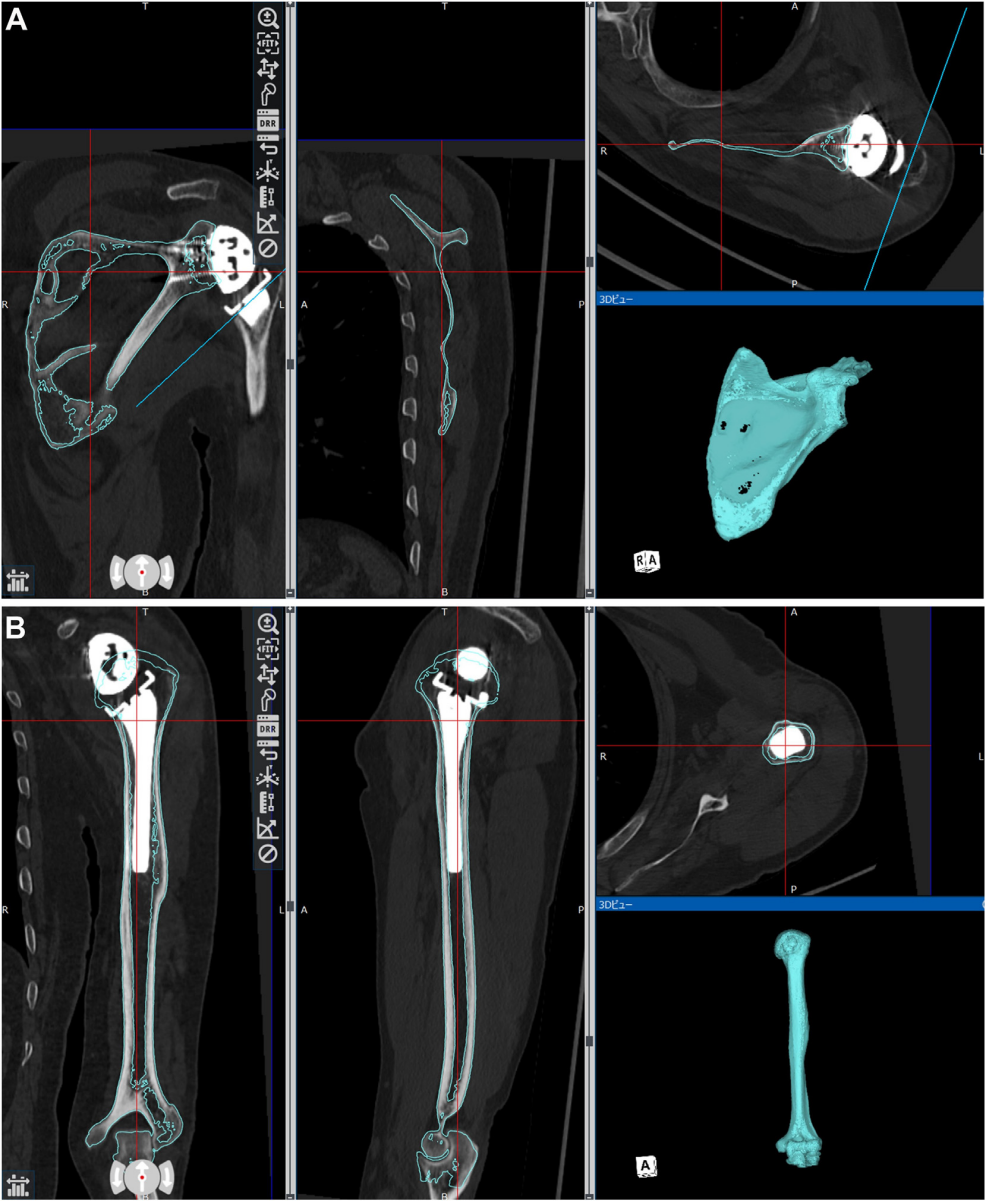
Method of superimposing postoperative computed tomography and preoperative data. A: Matching with the scapula. B: Matching with the humerus.
